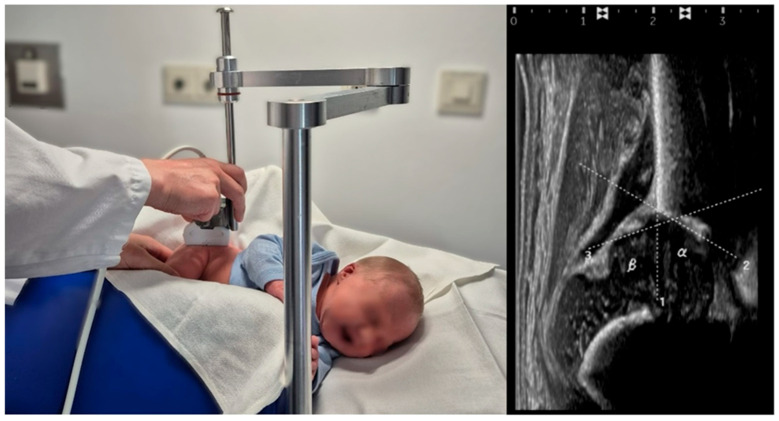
(Left): Representative image of the standardized ultrasound examination of a 2-day-old male neonate placed in a left lateral position using a positioning device, according to Graf. (Right): Sonographic assessment of the alpha and beta angle of the right hip was performed using the Graf method.
